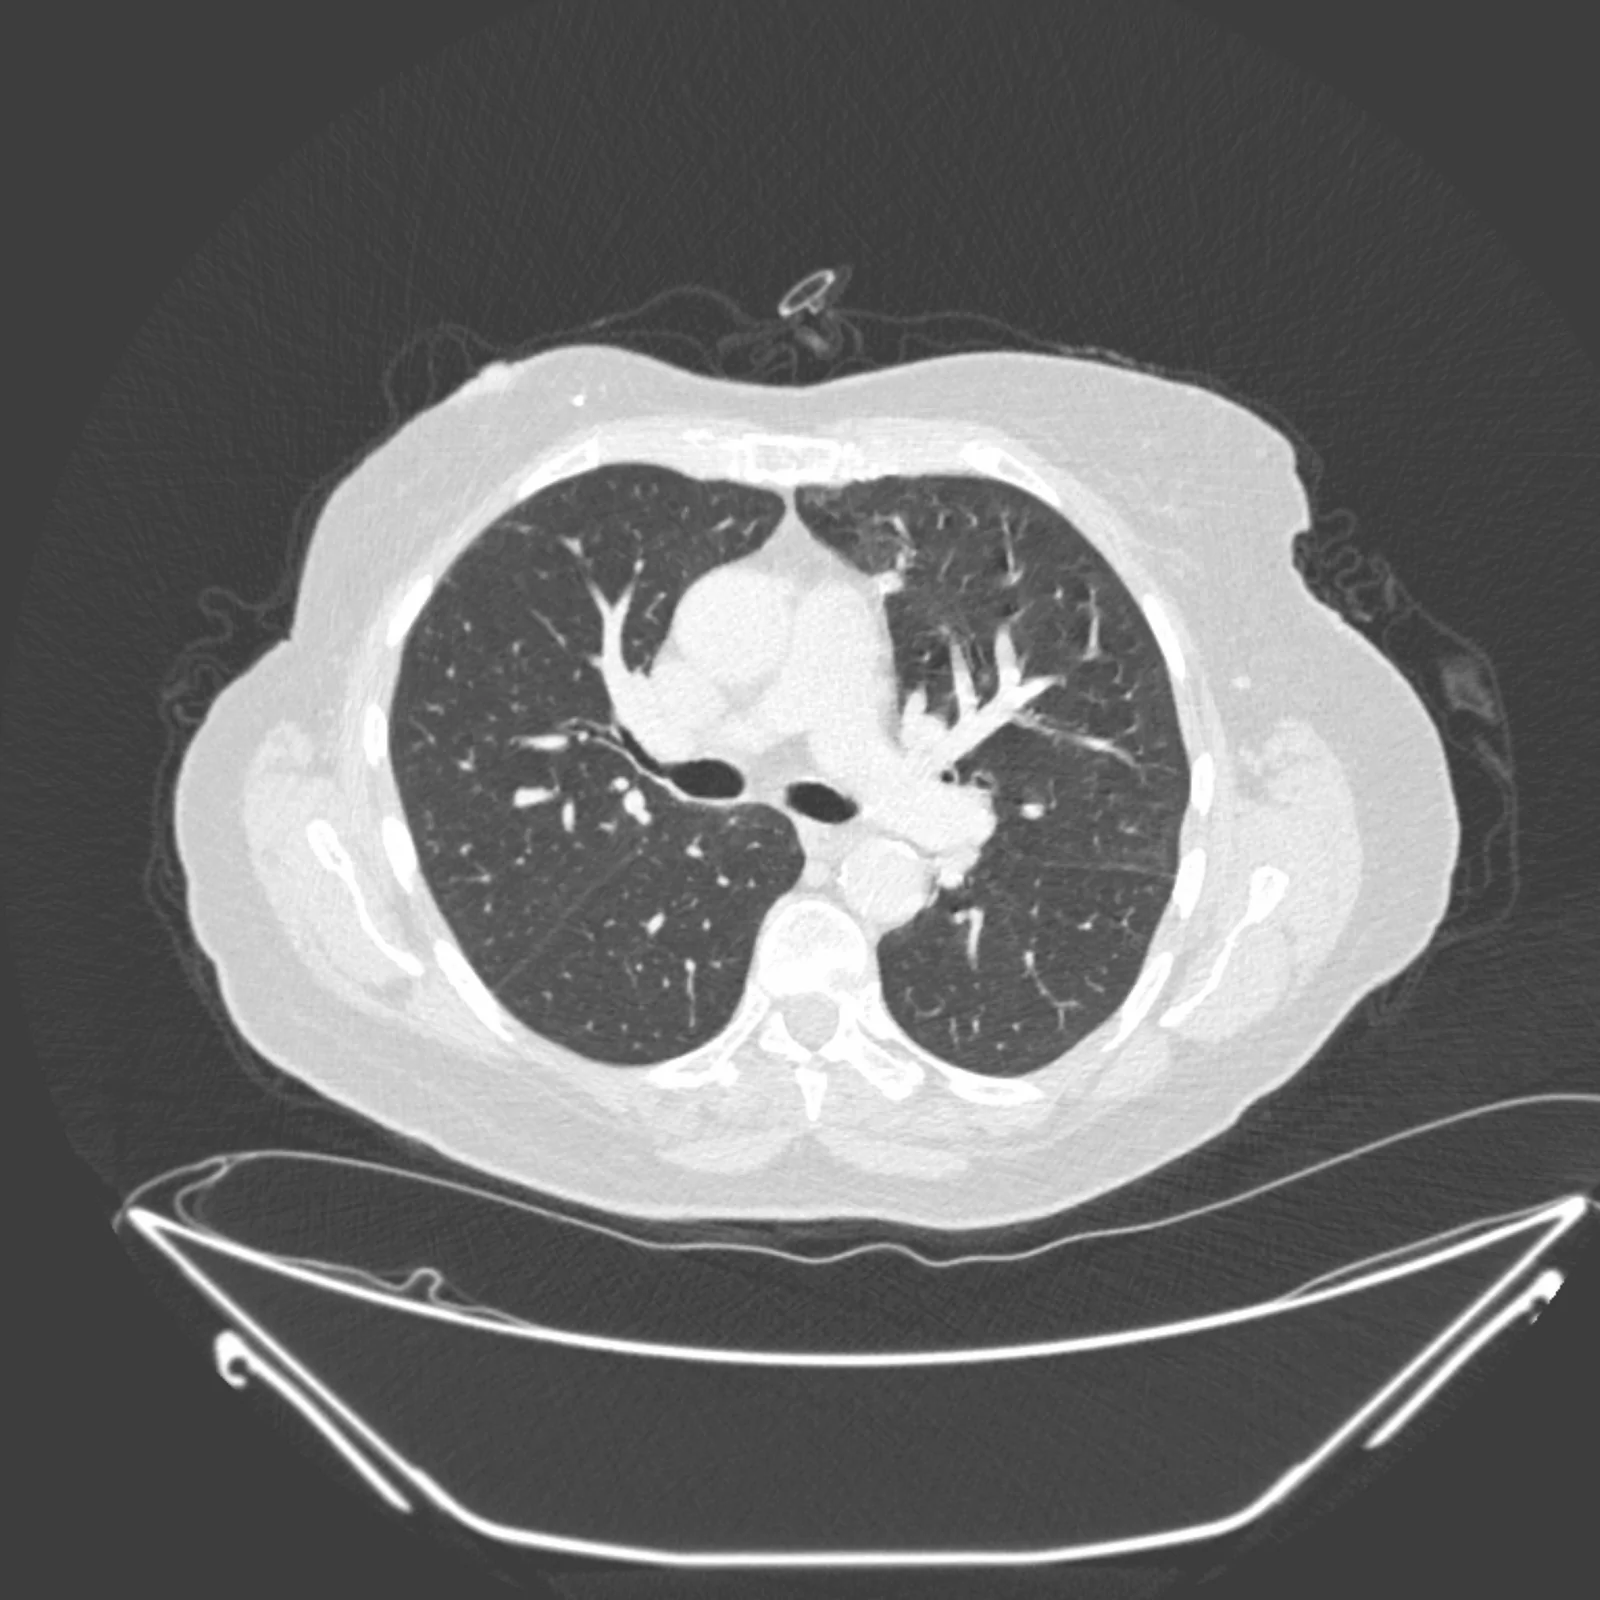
Cross-sectional CT image of the thorax showing no evidence of metastatic disease and good opacification of the pulmonary trunk.
